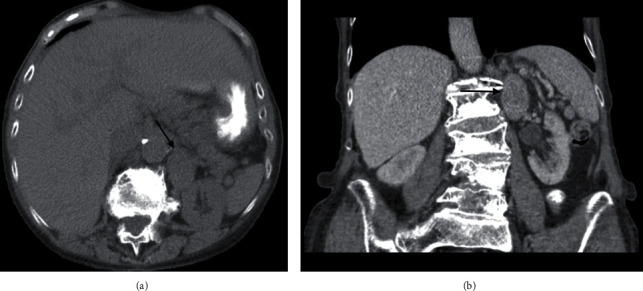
Initial CT abdomen showed a 3.3 cm left adrenal indeterminate mass.
